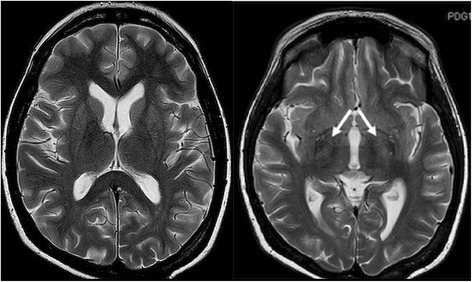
Symmetrical T2-hyperintensity of basal ganglia on MRI (white arrows)
